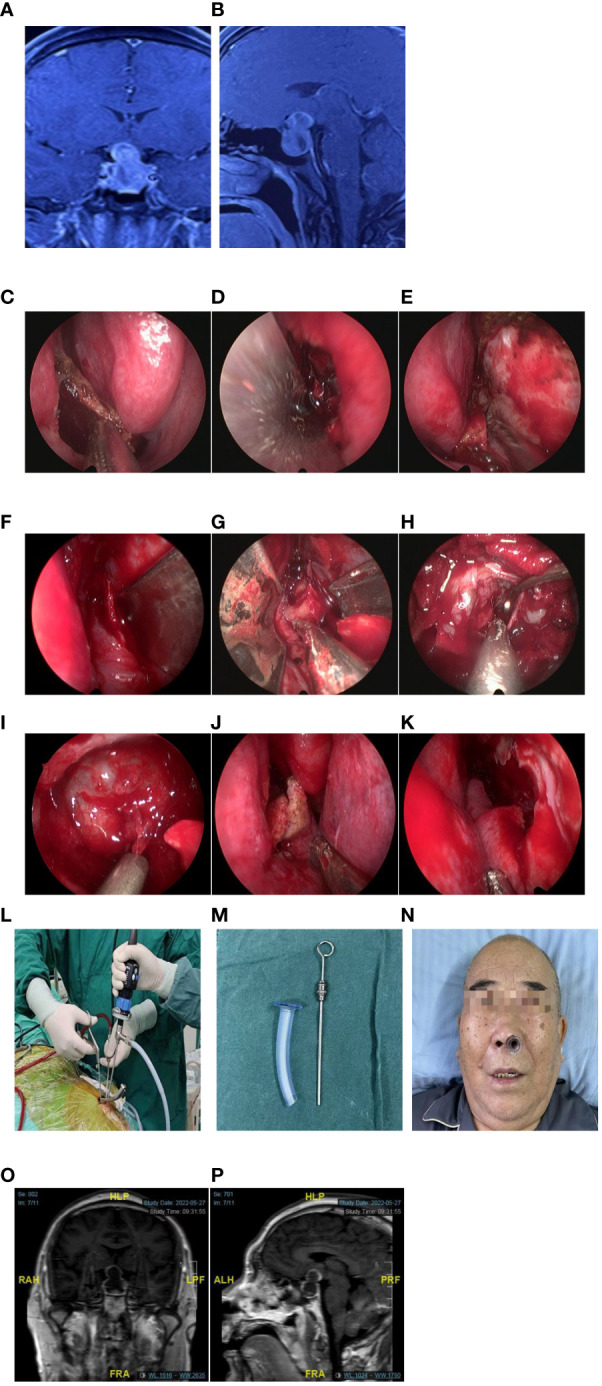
A case of giant pituitary adenoma (Knosp Grade 2) with bilateral cavernous sinus invasion and partial encasement of the internal carotid artery (ICA). [(A, B) T1-weighted magnetic resonance imaging (MRI) with gadolinium enhancement [(A) coronal section, (B): sagittal section]. (C) Rescue Nasoseptal flap (NSF) in the right nasal cavity. (D) Incision made in the left nasal septal mucosa. (E, F) The right and left blades were separately introduced into the respective nasal cavities, aided by brain retractors. (G) Creation of a surgical pathway by expanding the binasal speculum. (H) Tumor resection. (I) The saddle diaphragm drop. (J, K) Preservation of nasal anatomy in the right and left postoperative nasalcavities.Intraoperatively, the surgeon manipulated bimanually free under the corridor, while the assistant was holding the endoscope (L). (M, N) A shorter nasopharyngeal airway device is inserted in the left nostril to ensure postoperative ventilation and enhance patient comfort. (O, P) Postoperative T1-weighted MRI with gadolinium enhancement: gross total removal of the tumor [(O): coronal sections, (P): sagittal section].
